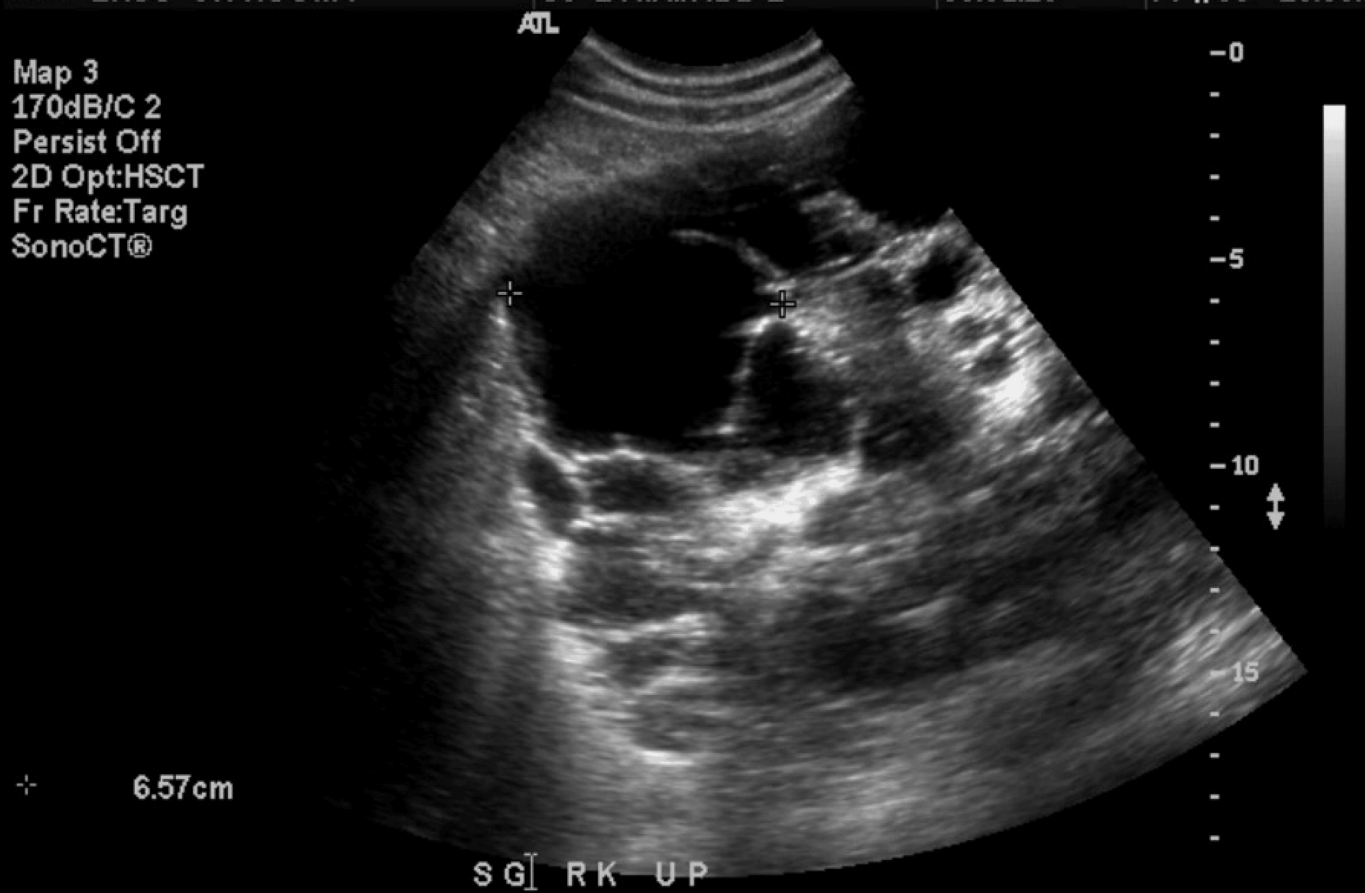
Polycystic kidney disease on ultrasound in the right kidney with a large cyst measuring 6.6 cm.
